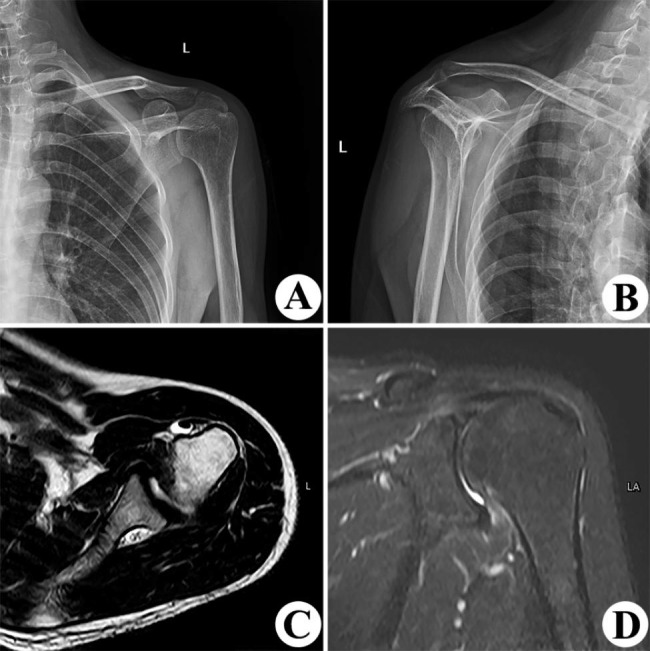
This was a 48-year-old man who presented with recurrent left shoulder pain for more than 2 years diagnosed with bursal-side Ellman grade III PTRCTs. (A) Anterior-posterior X-ray of the left shoulder. (B) X-ray of the left shoulder in the Y-view. (C) MRI of the long head of the biceps tendon (D) T2-weighted coronal MRI sequence with bursal-side Ellman grade III PTRCTs.
